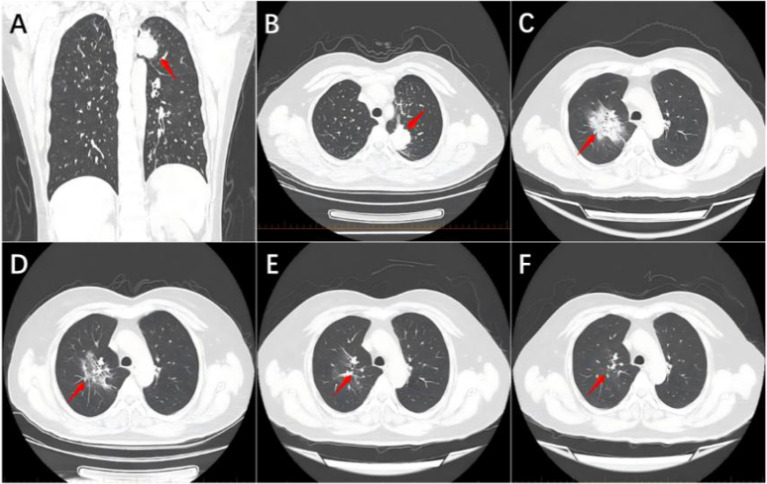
Series of chest CT images. (A) Coronal image from March 3, 2022, showing a mass approximately 3.1 cm × 2.9 cm in the left upper lobe (red arrow). (B) Axial image from March 3, 2022, showing the same mass in the left upper lobe (red arrow). (C) Image from April 14, 2025, showing a newly developed patchy high-density opacity approximately 5.4 cm × 3.7 cm in the right lung (red arrow). (D) Image from May 15, 2025, showing a persistent patchy focus in the right lung (red arrow). (E) Image from July 7, 2025, showing a patchy shadow in the right lung (red arrow). (F) Image from October 9, 2025, showing a patchy shadow in the right lung (red arrow).
